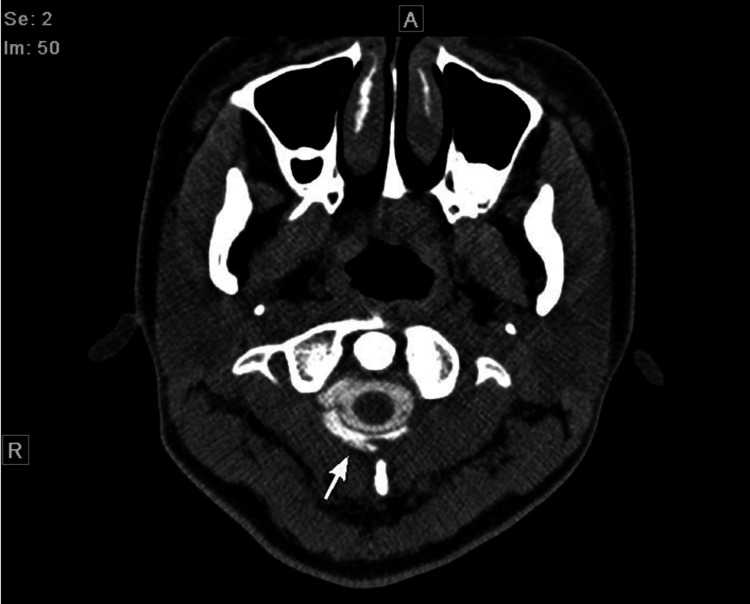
Contrast-enhanced computed tomography of the spinal cord shows extravasation of contrast into the epidural space
